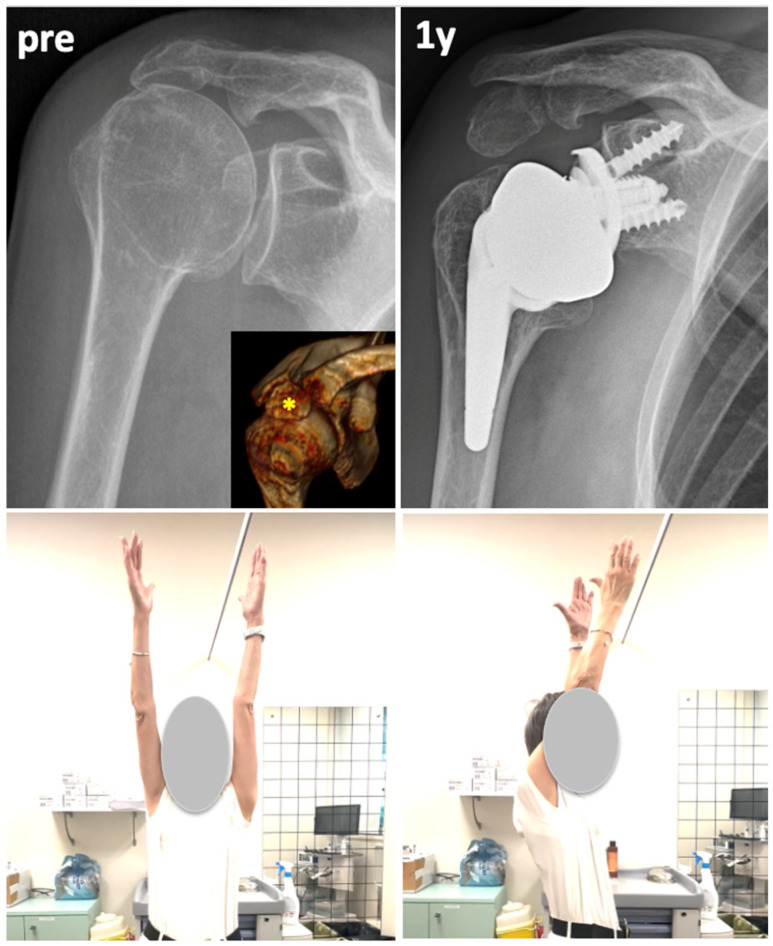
A case of RTSA in case of os acromiale (yellow * on the 3D CT scan). After 1 year from RTSA, the x-ray shows an evident inferior tilt of the meso-acromion with decreased acromiohumeral distance. Clinically, the patient recover good abduction and anterior flexion.
